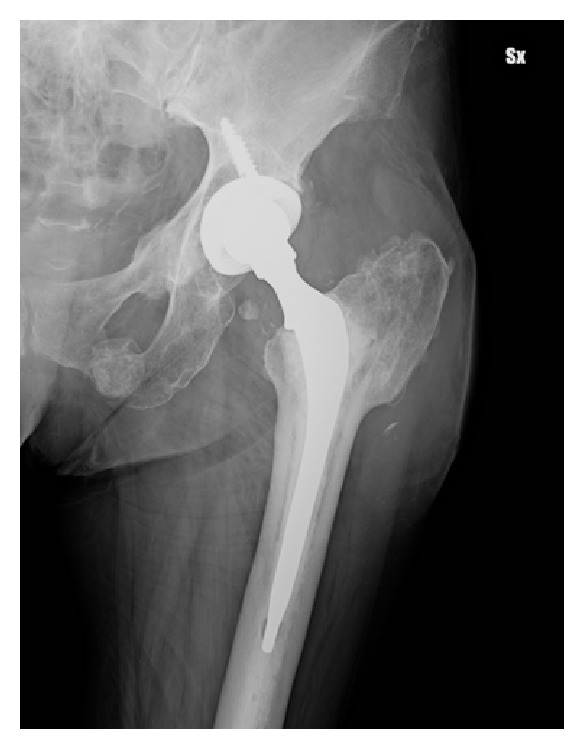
Preoperative X-ray total hip arthroplasty.
